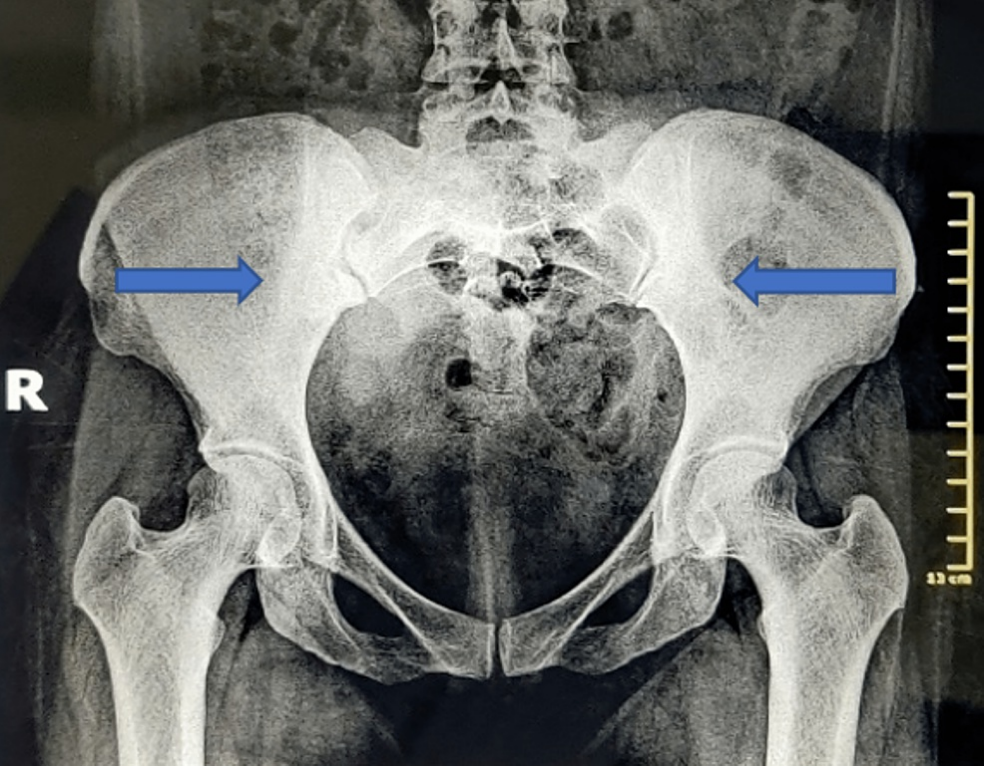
Antero-posterior radiograph of pelvis showing remarkable sclerosis at the iliac border of the bilateral sacroiliac joints.
